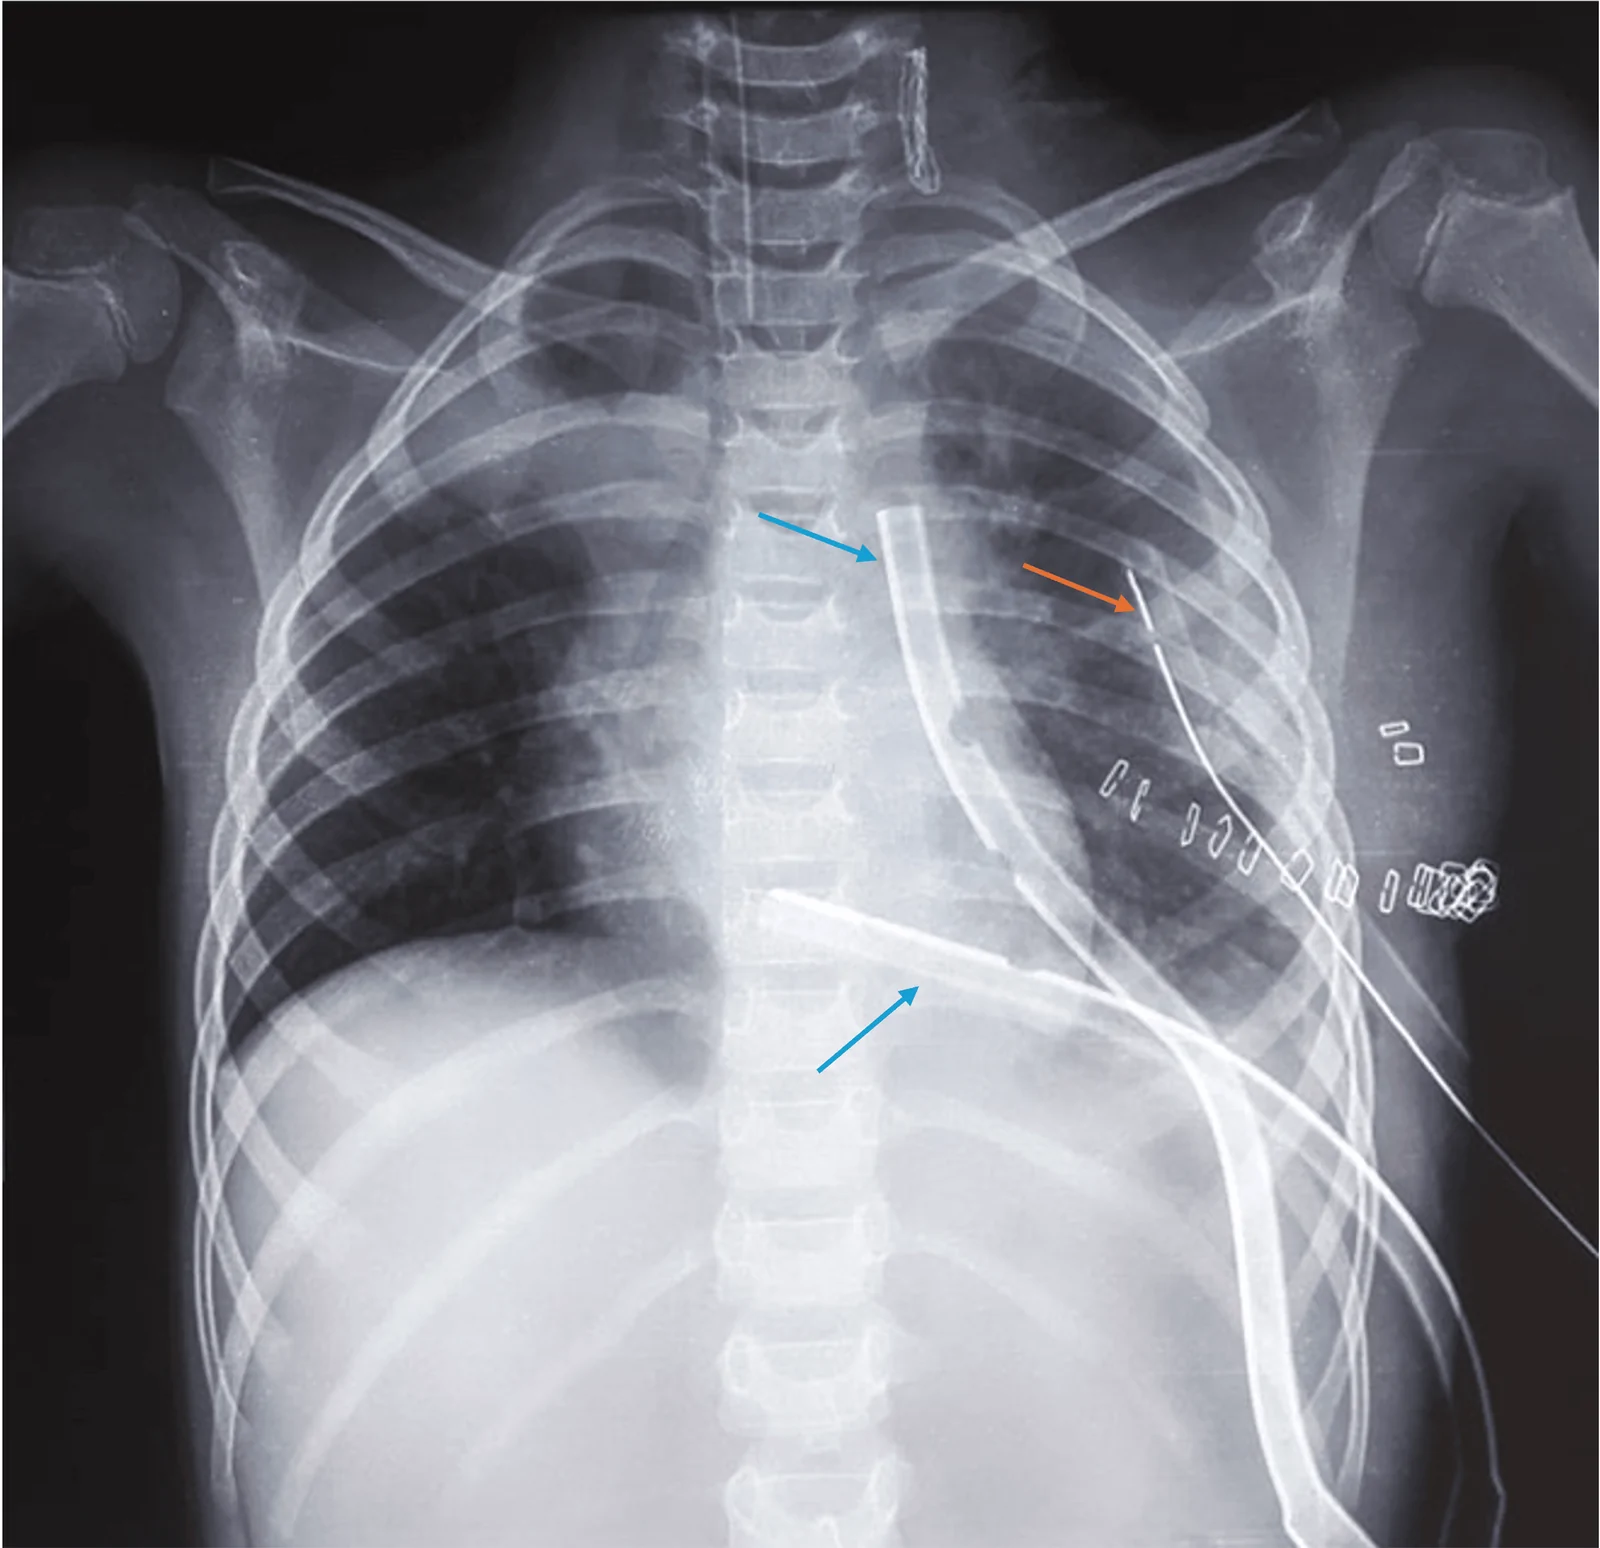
Postoperative chest radiograph showing one left thoracic drain (orange arrow) and two mediastinal drains (blue arrows).
